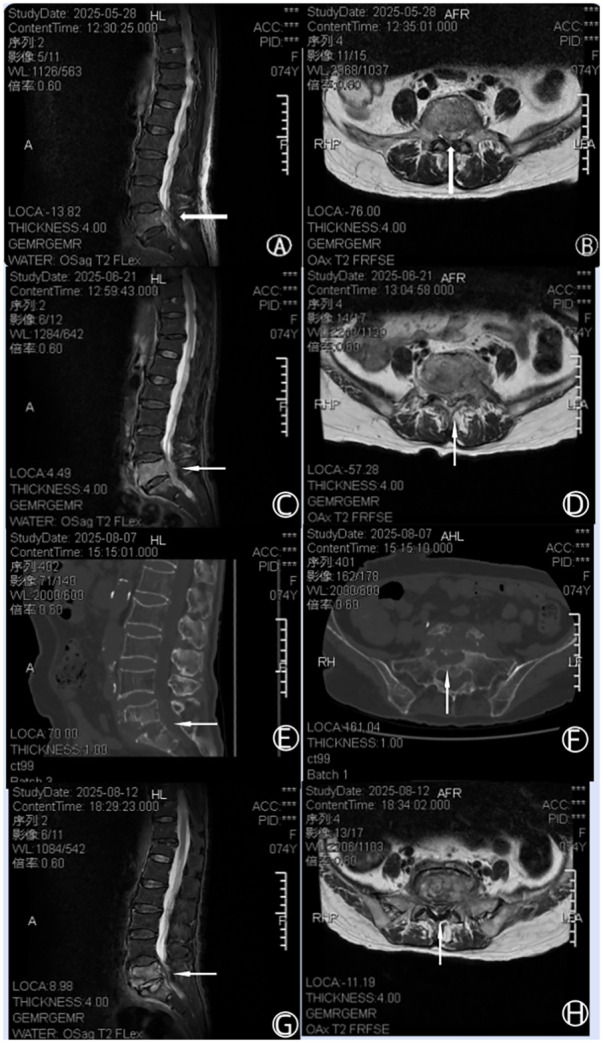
Preoperative lumbar MRI and CT showing progression of the infection at the L5 vertebra with epidural abscess formation.
